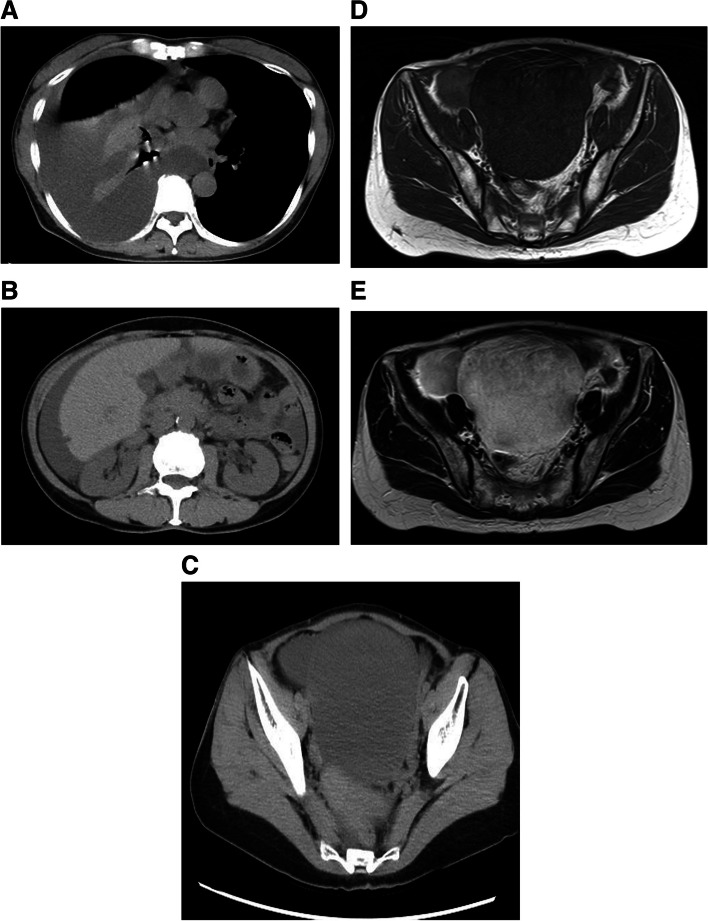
CT. A Chest: pleural effusion, passive atelectasis. B, C Abdomen and pelvis: ascites and a huge tumor 10 cm in diameter in the pelvis. MRI. D Hypointense signals on T1-weighted MRI. E Hyperintense signals on T2-weighted MRI
